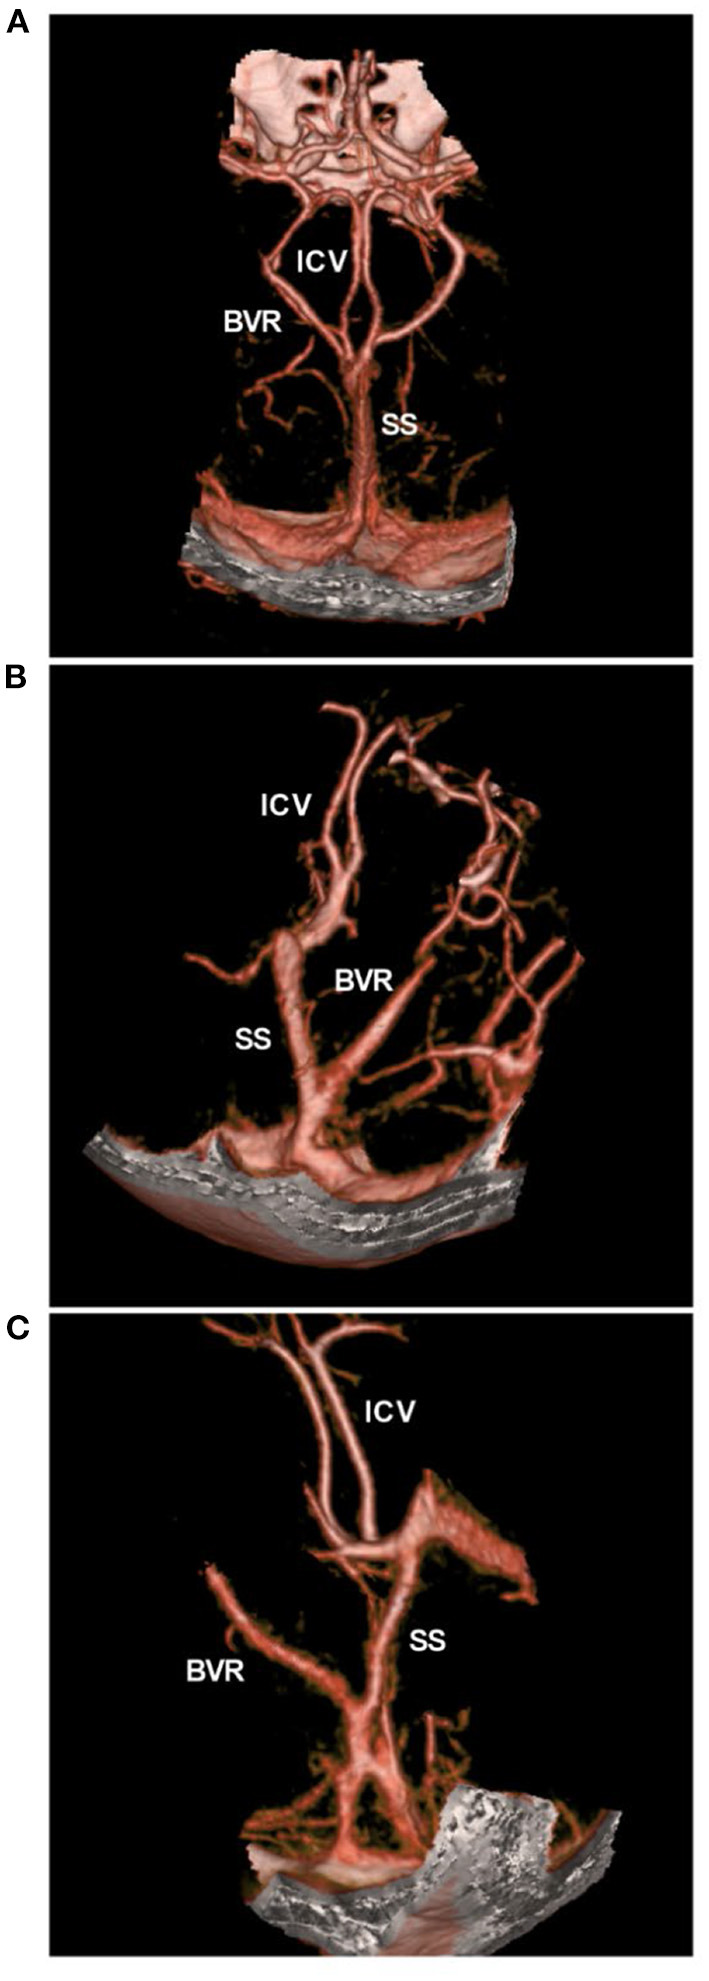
BVR variants in CTA. (A) CTA showing the normal pattern of deep veins, bilateral ICVs and BVRs that flow into the vein of Galen. (B,C) CTA showing the BVR that flowed into the straight sinus. BVR, Basal vein of Rosenthal; CTA, computed tomography angiography; ICV, internal cerebral vein, SS, straight sinus.
